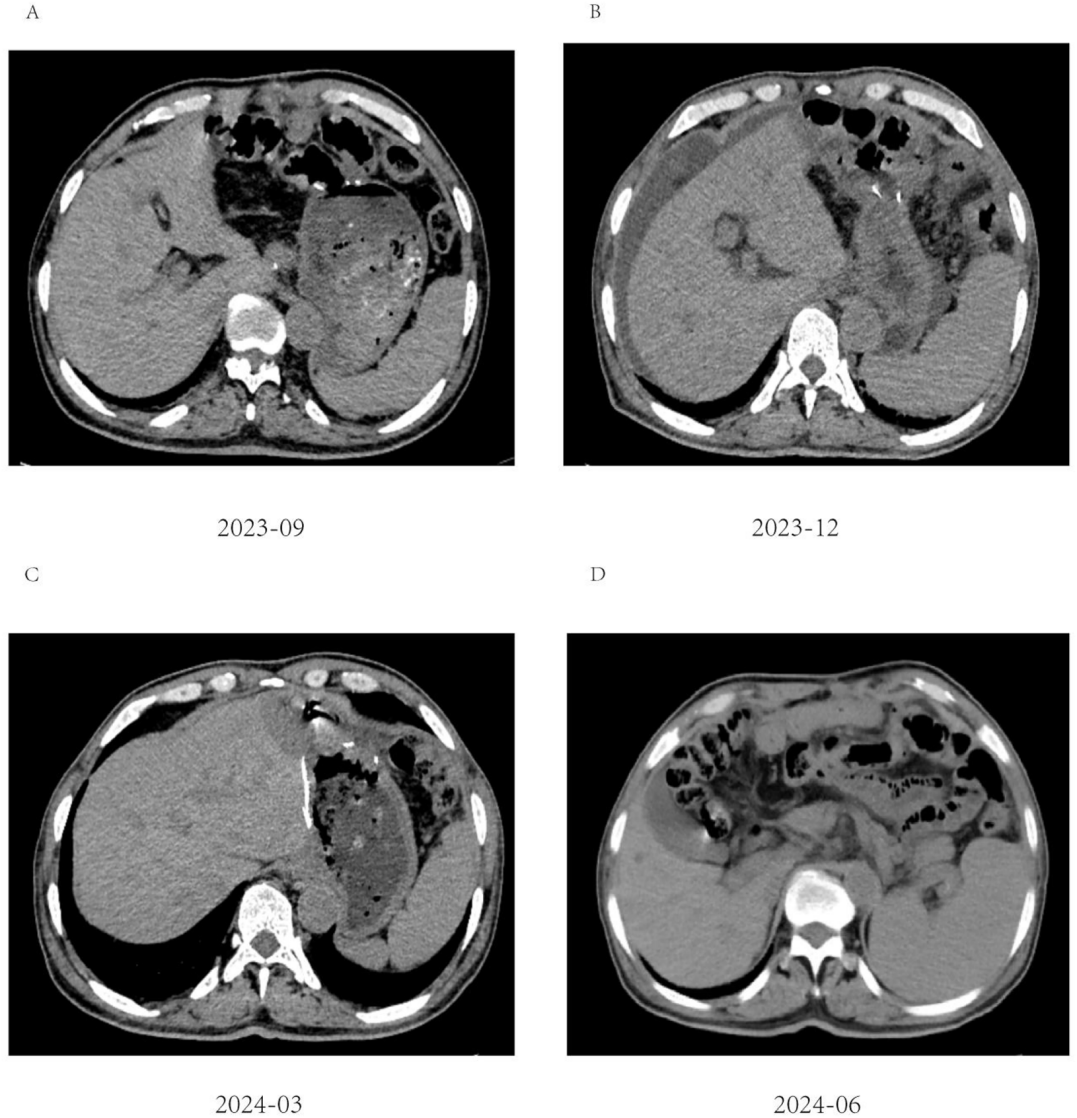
Abdominal CT imaging during follow-up. (A-D) Postoperative CT showed that the patient’s condition was stable, and no tumor recurrence was found; (B) Unexplained abdominal effusion was detected in 2023-12, with no accompanying discomfort. No special treatment was administered, and a review after 3 months showed that the peritoneal effusion had disappeared.
